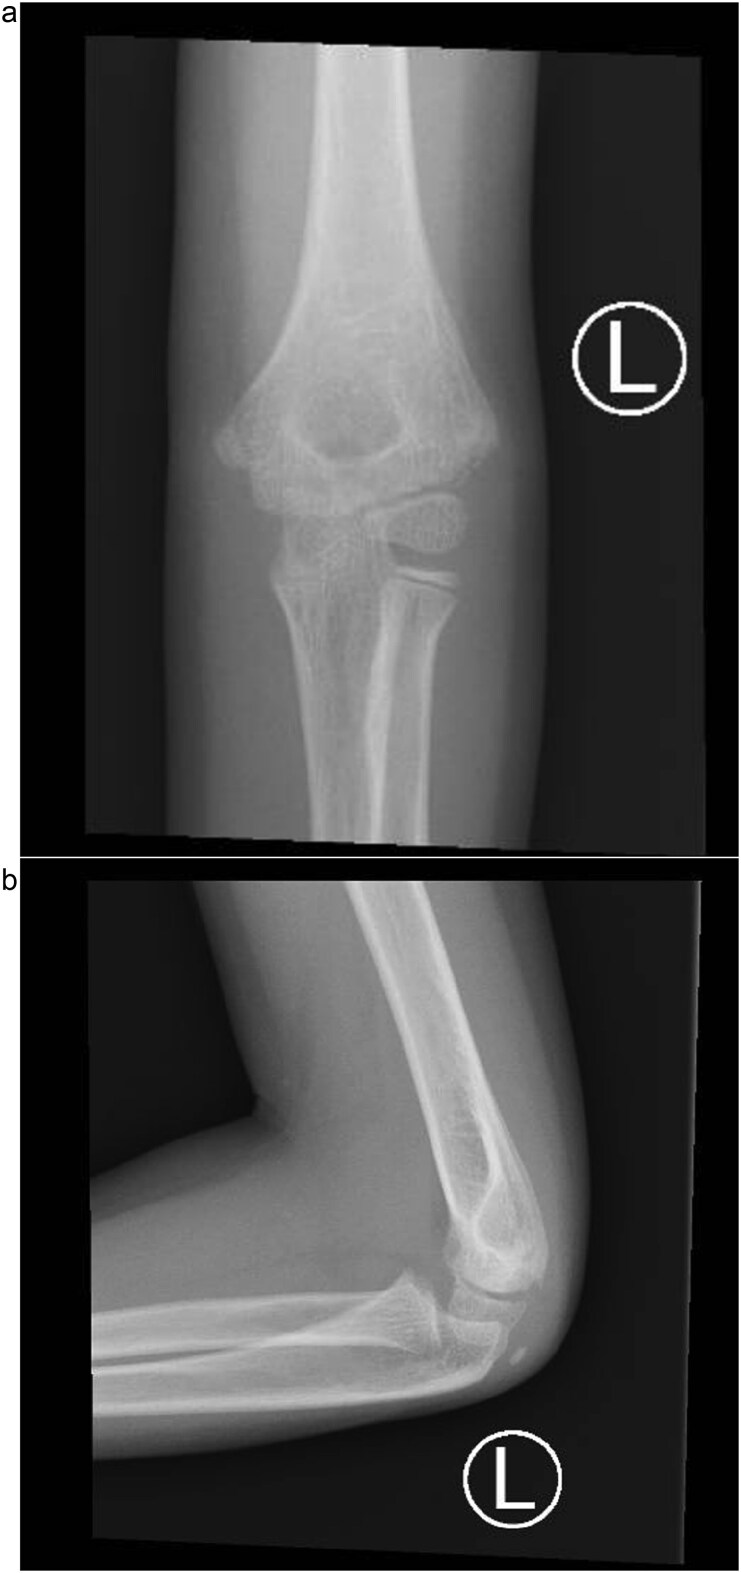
(a) Six-month follow-up anteroposterior radiograph showing complete union of the lateral condyle fracture after Kirschner wire removal. (b) Six-month lateral radiograph demonstrating full healing with restored joint alignment, maintained carrying angle, and no evidence of growth disturbance or deformity.
